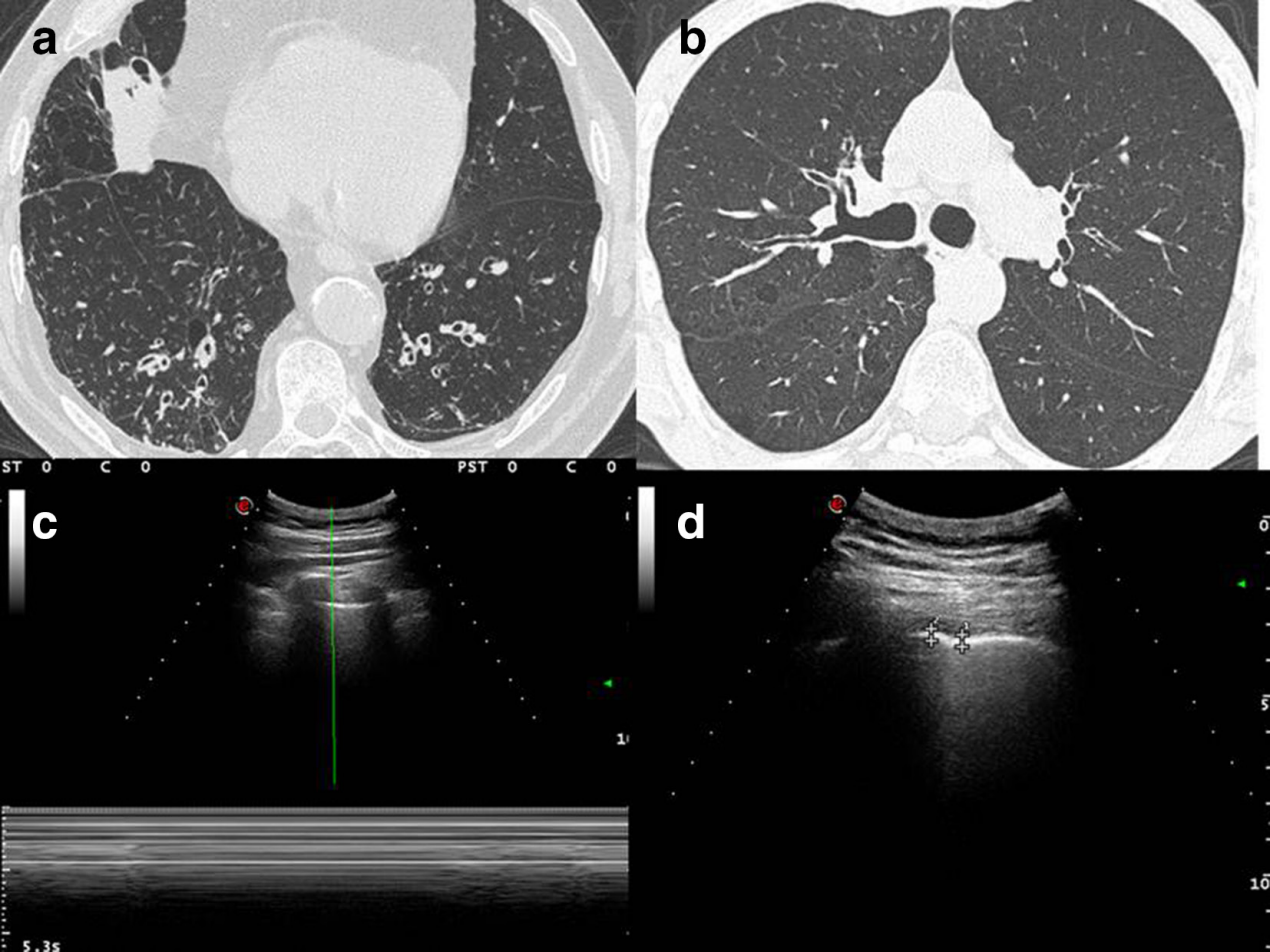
(A, B) HRCT imaging shows overdistension and the airway wall thickening with bronchiectasis of lung bases; in addition, it shows also centrilobular micronodular lesions which state distal airway inflammation. (C) Ultrasound imaging, taken with convex probe (5 MHz), indicates the absence of gliding sign, which was confirmed by Barcode sign in M-mode. (D) Ultrasound imaging, with convex probe (5 MHz) shows an irregular thickening of the hyperechoic pleural line (3.1–3.6 mm). HRCT, high resolution CT.
